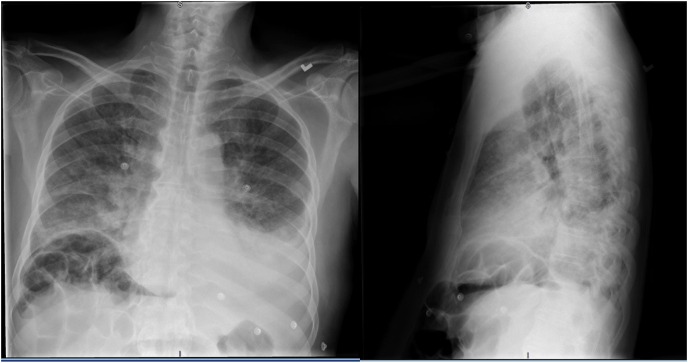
Posteroanterior 2-view chest radiograph on 5/10/21: diffuse extensive bilateral airspace infiltrates remain with possible associated effusions more prominently in the left. Heart, mediastinum, and osseous structures are intact. Interposition of the right hepatic flexure above the liver above the hemidiaphragm is demonstrated (Chilaiditi's sign).
